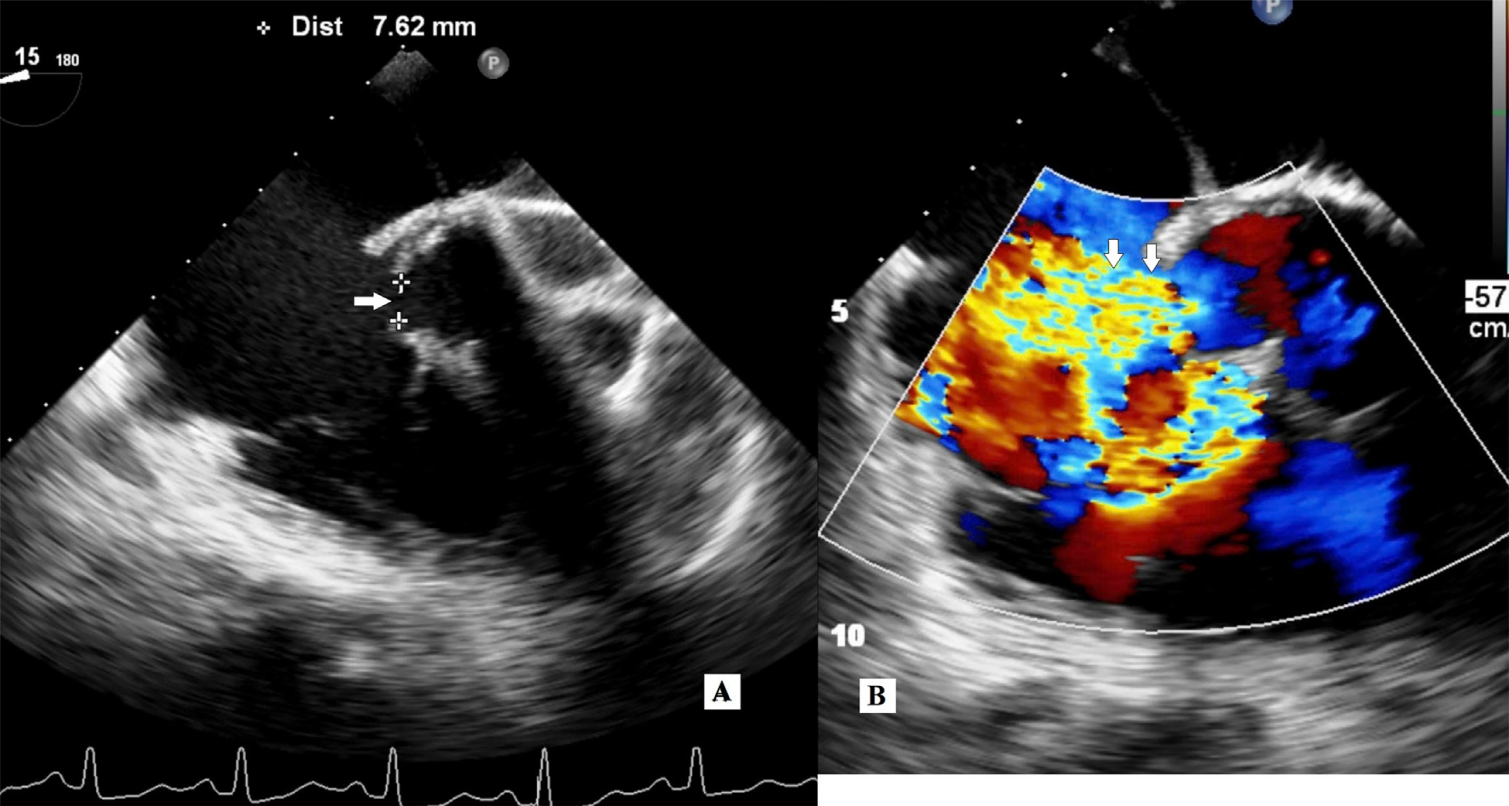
TEE in the first patient. (A) Mid-esophageal long-axis 2D view (∼0–15°) shows a rupture in the membranous portion of the atrioventricular septum, forming a direct LV-RA shunt. The defect measures 7.6 mm (calipers) and is located superior to the tricuspid valve septal leaflet (white arrow). (B) Color Doppler in the same view demonstrates a high-velocity left-to-right jet with turbulent flow entering the right atrium posterior to the tricuspid valve, consistent with a direct (supravalvular) Gerbode-type defect (white arrow). Velocity scale: ±57.4 cm/s.
